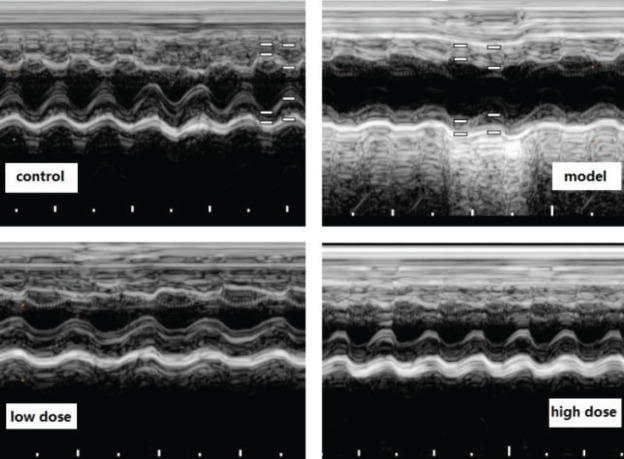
Typical pictures of echocardiography results for the *in vivo* study
